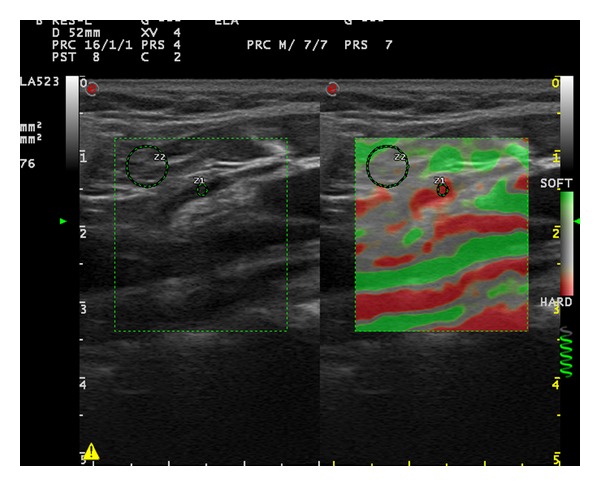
Elastosonography of the cervical lymph node on the right side of a 55-year-old female patient (long axis: 19.8 mm, short axis: 4.9 mm, cortical thickness: 1.7 mm, hilar thickness: 3.1 mm, strain ratio: 3.76, and elasticity score: 3).
